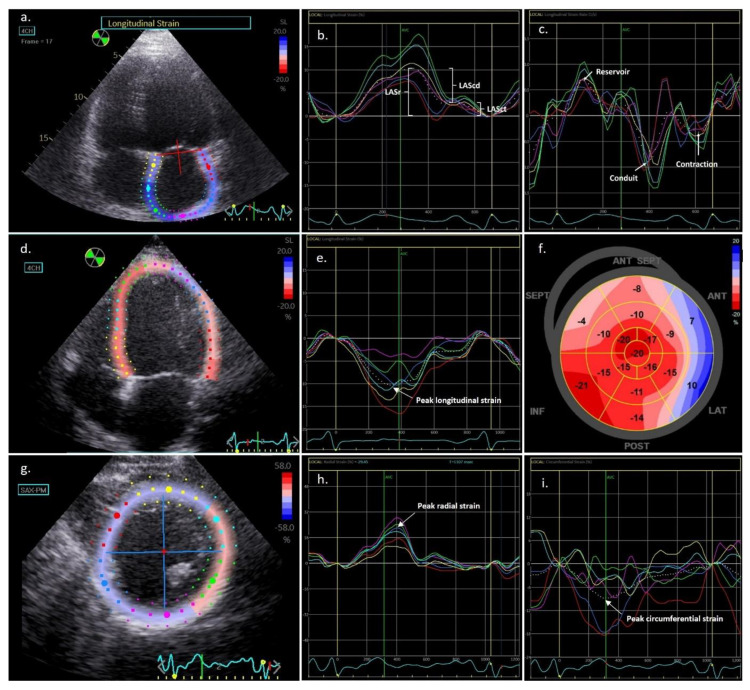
Left atrial and ventricular strain analysis via speckle tracking echocardiography. (a) Measurement of left atrial strain, semi-automatic contouring of the left atrial borders for speckle tracking; (b) Display of the left atrial deformation over a cardiac cycle starting at the QRS, with the 3 phases: reservoir (LASr), conduit (LAScd), and contractile (LASct) function; (c) Strain rate measurements in the reservoir, conduit, and contraction phases; (d) Measurement of left ventricular (LV) longitudinal strain, semi-automatic contouring of the endocardial borders for speckle tracking; (e) Display of the LV longitudinal strain over a cardiac cycle, with the arrow showing the peak longitudinal strain, (f) Global longitudinal strain bull’s eye plot in a patient with ischemic heart failure with reduced ejection fraction and basal antero-lateral hypokinesia; (g) Measurement of LV radial and circumferential strain with semi-automatic contouring; (h) Display of the LV radial strain over a cardiac cycle, with the arrow showing the peak longitudinal strain; (i) Display of the LV circumferential strain over a cardiac cycle, with the arrow showing the peak longitudinal strain.
